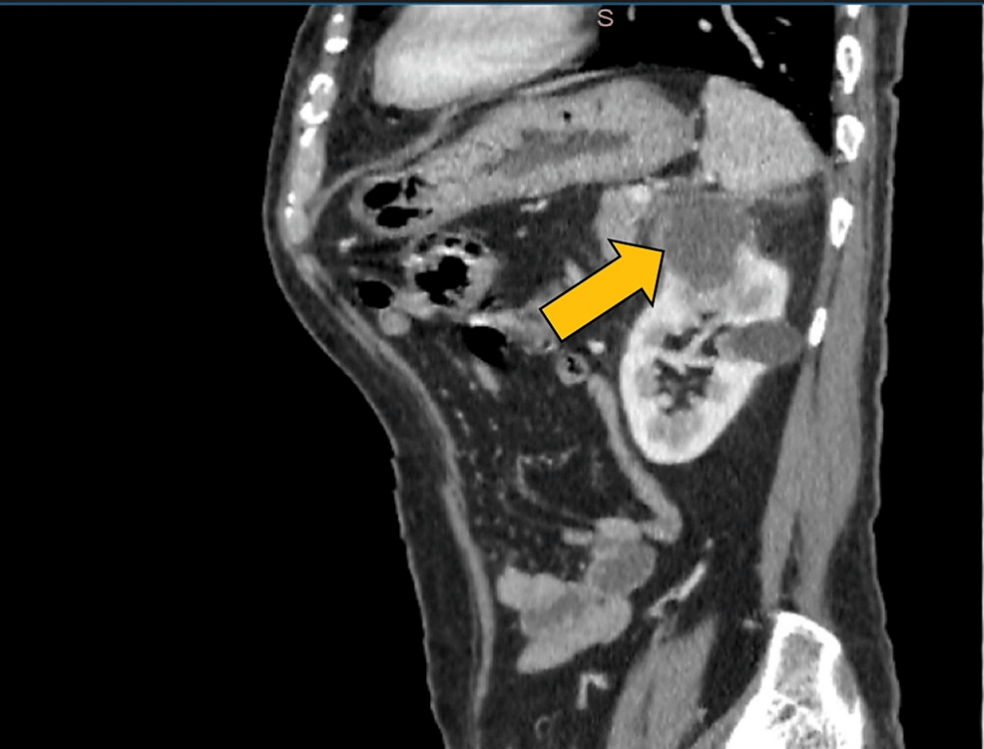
Sagittal CECT showing a relatively well-defined mass of low attenuation with a thick irregular enhancing wall or pseudocapsule at the upper pole of the left kidney CECT: Contrast-enhanced computed tomography.
